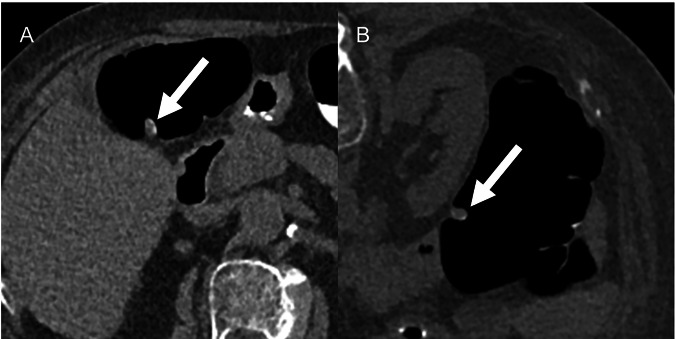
Supine (A) and prone (B) CT-colonography images in a 77-year-old female reveal a 8 mm polypoid lesion in the transverse colon. The lesion is classified as C-RADS C2a
